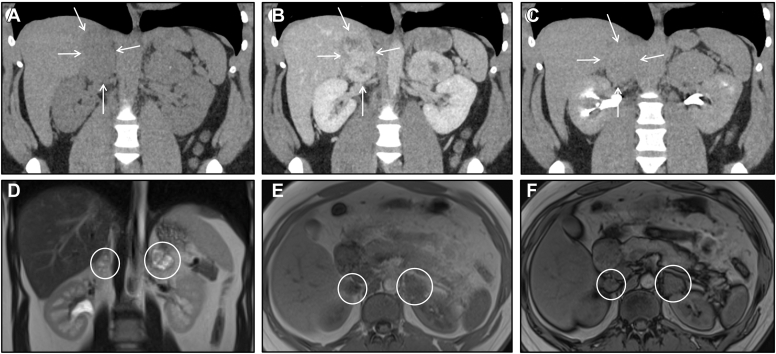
Bilateral pheochromocytomas in Von-Hippel Lindau syndrome. (*A*-*C*) Coronal adrenal-protocol CT before contrast (*A*), in the 75-second-delay phase (*B*), and in the 15-minute-delay phase (*C*) demonstrate bilateral heterogeneously hyperenhancing adrenal masses, *right* greater than *left* (white arrows) consistent with bilateral pheochromocytomas in the setting of Von-Hippel Lindau syndrome. (*D*-*F*) Coronal T2 image (*D*) demonstrate bilateral heterogeneously hyperintense T2 lesions, in keeping with classic light-bulb sign on T2 for pheochromocytomas (circles). Axial in-phase (*E*) and opposed-phase (*F*) images demonstrate no change in signal, in contrast to adenomas in Fig. 1. *CT* = computed tomography.
